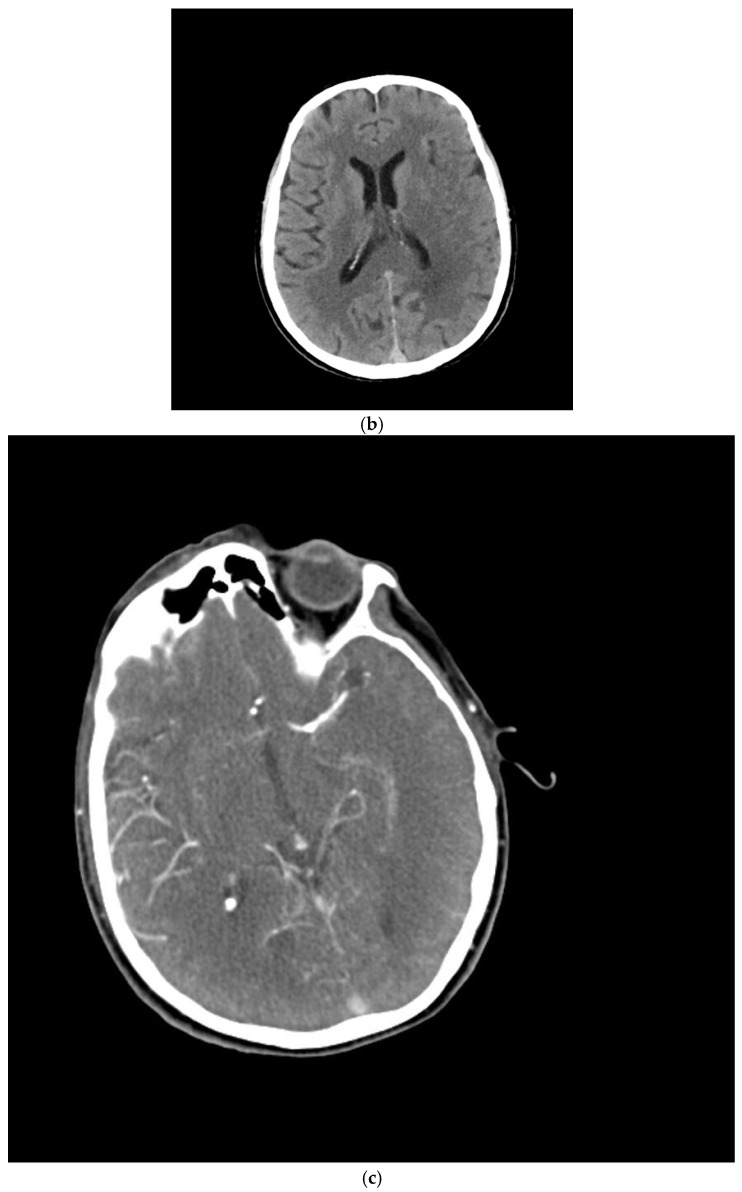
(a) ©DWI in a 73-year-old male patient; hypointense acute diffusion limitation in ADC affecting most of the left cerebral hemisphere; (b) faint hypodense area in the left cerebral hemisphere on non-contrast brain CT; (c) contrast-enhanced brain CT angiography shows left MCA M1 occluded from distal onwards.
